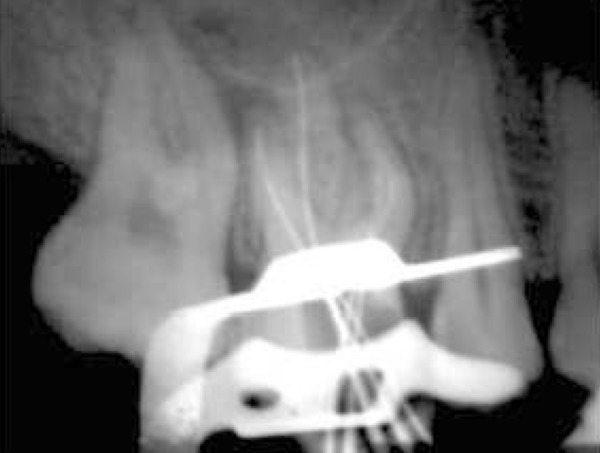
The working length radiograph of tooth #16
